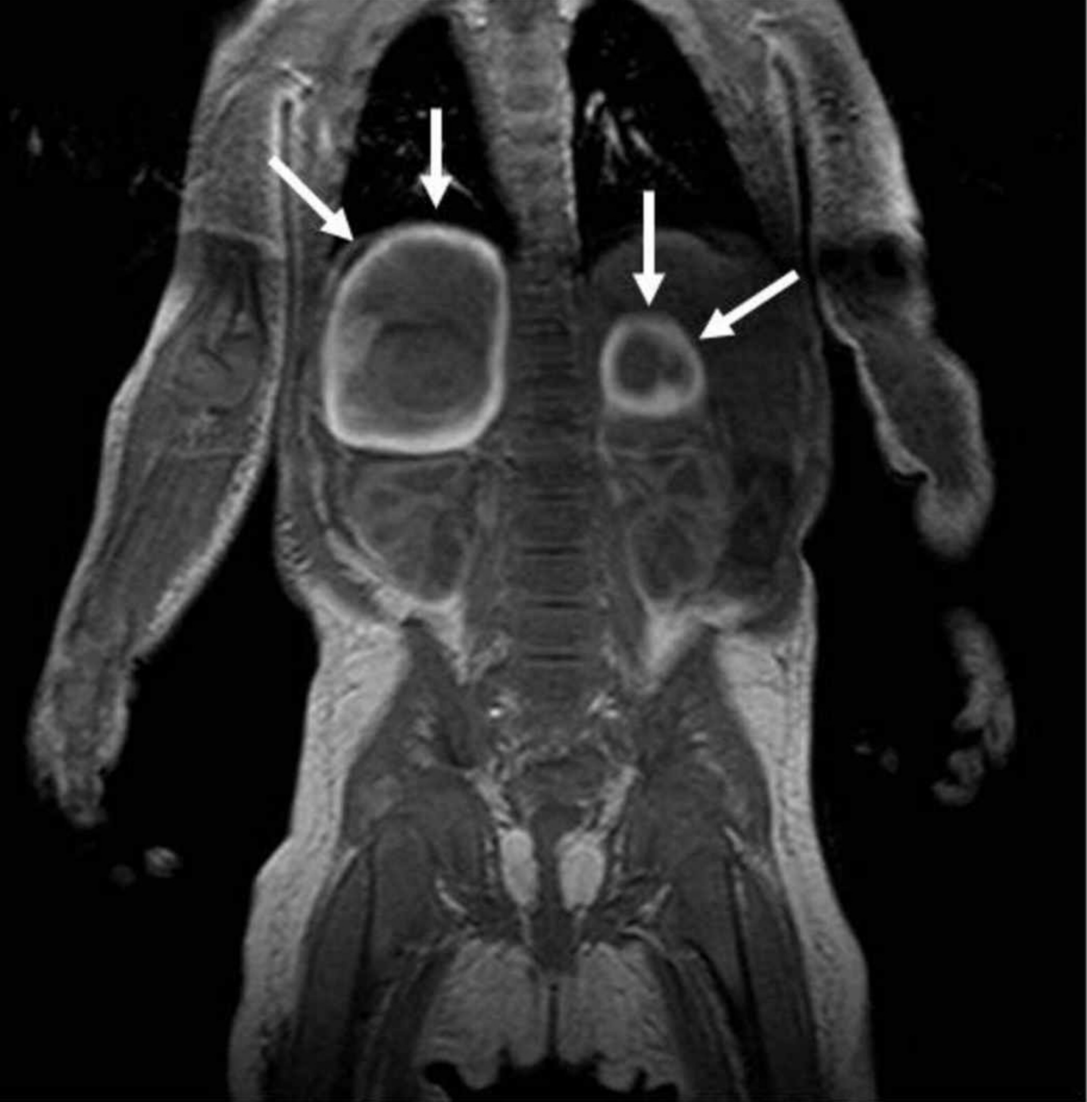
Bilateral adrenal cystic masses with hyperintense hemorrhagic changes are seen on coronal T1-weighted image (arrows)
